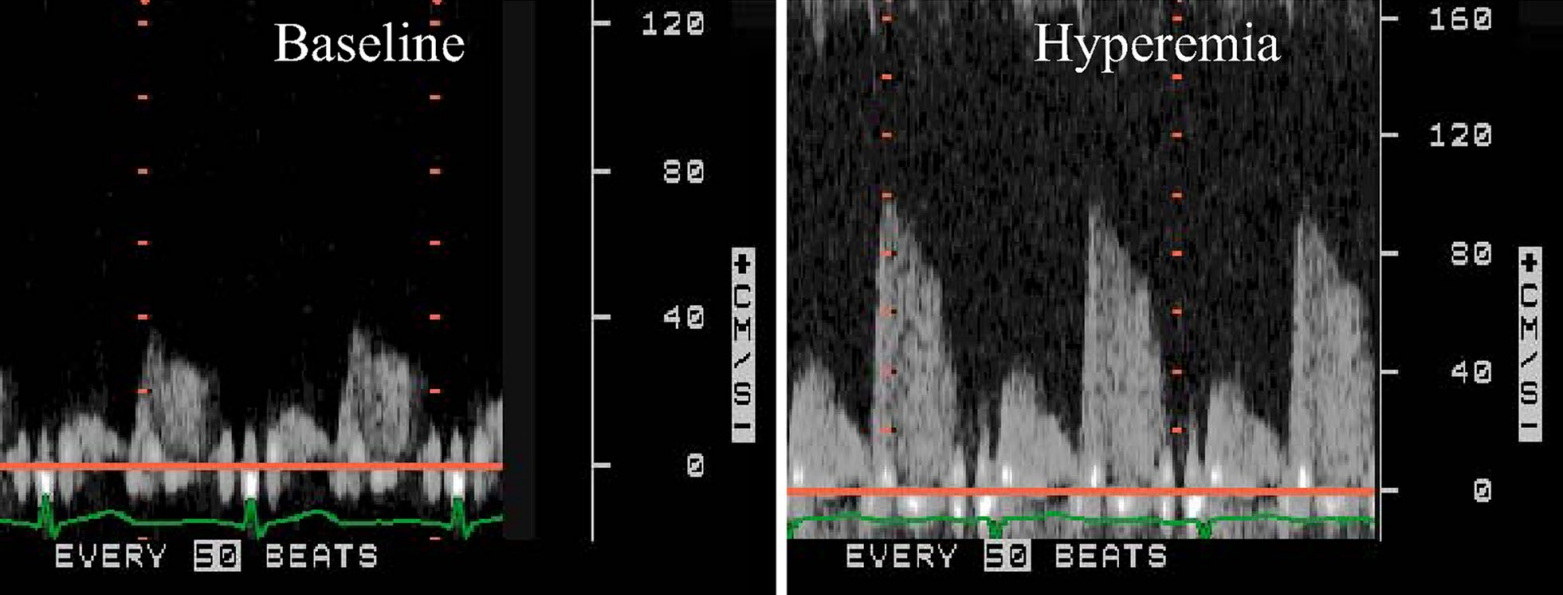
Example of Doppler tracing of LAD blood flow during baseline and after adenosine injection (hyperemia).
